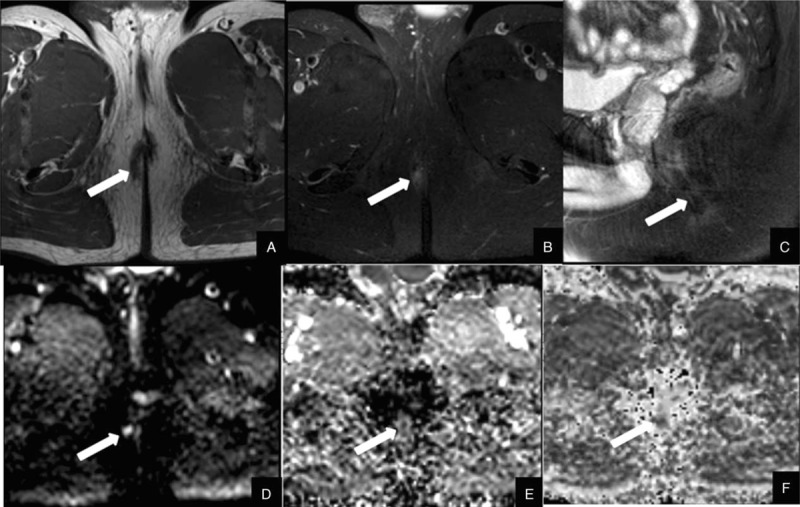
A 39-year-old man with subcutaneous anal fistula of negative inflammation activity. (A) Axial T2-weighted image shows a perianal fistula (arrow) that exhibits equal and slightly high signal intensity. (B and C) Axial and sagittal fat-suppression T2-weighted images show a perianal fistula (arrow) that exhibits high signal intensity. (D) Axial diffusion tensor imaging reveals high signal intensity at the fistula (arrow). (E) Axial apparent diffusion coefficient (ADC) map shows a value of 1.149 × 10^−3^ mm^2^/s for the fistula. (F) Axial fractional anisotropy (FA) map shows a value of 0.13.
